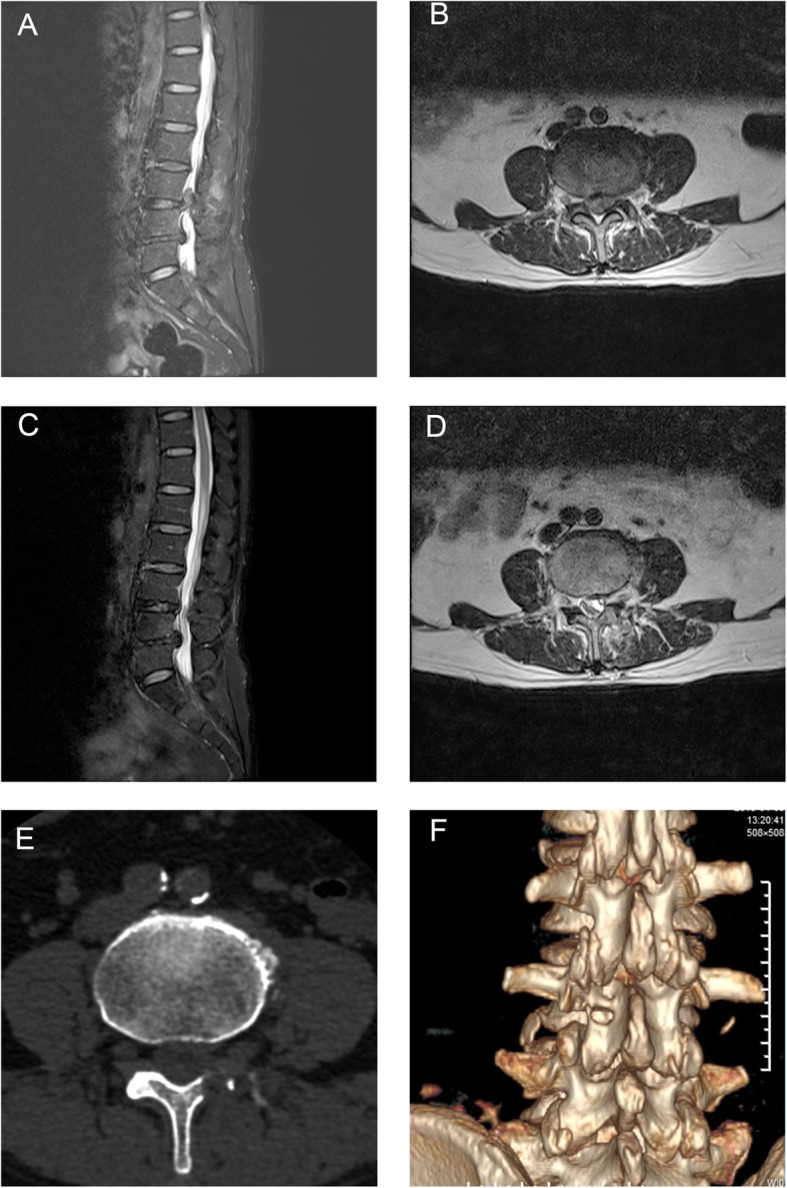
Case 2: a 62-year-old woman was admitted to the hospital because of “low back pain with pain in the medial aspect of the left lower leg for 2 months”. Lumbar MRI showed that the L3/4 disc had migrated to zone 1 in the posterior right upper side of the body, which was accompanied by L4/5 disc herniation (Fig. 7a, b). According to the patient’s signs and imaging examination, we determined that the patient’s symptoms were caused by L4 nerve root compression. Therefore, the patient underwent posterior PELD to remove the L3/4 upward migrated herniated disc. MRI was immediately repeated, which showed that the protruding tissue in the spinal canal was completely removed and that the spinal canal was re-expanded (Fig. 7c, d). Postoperative CT showed the bone tunnel (Fig. 7e, f)
